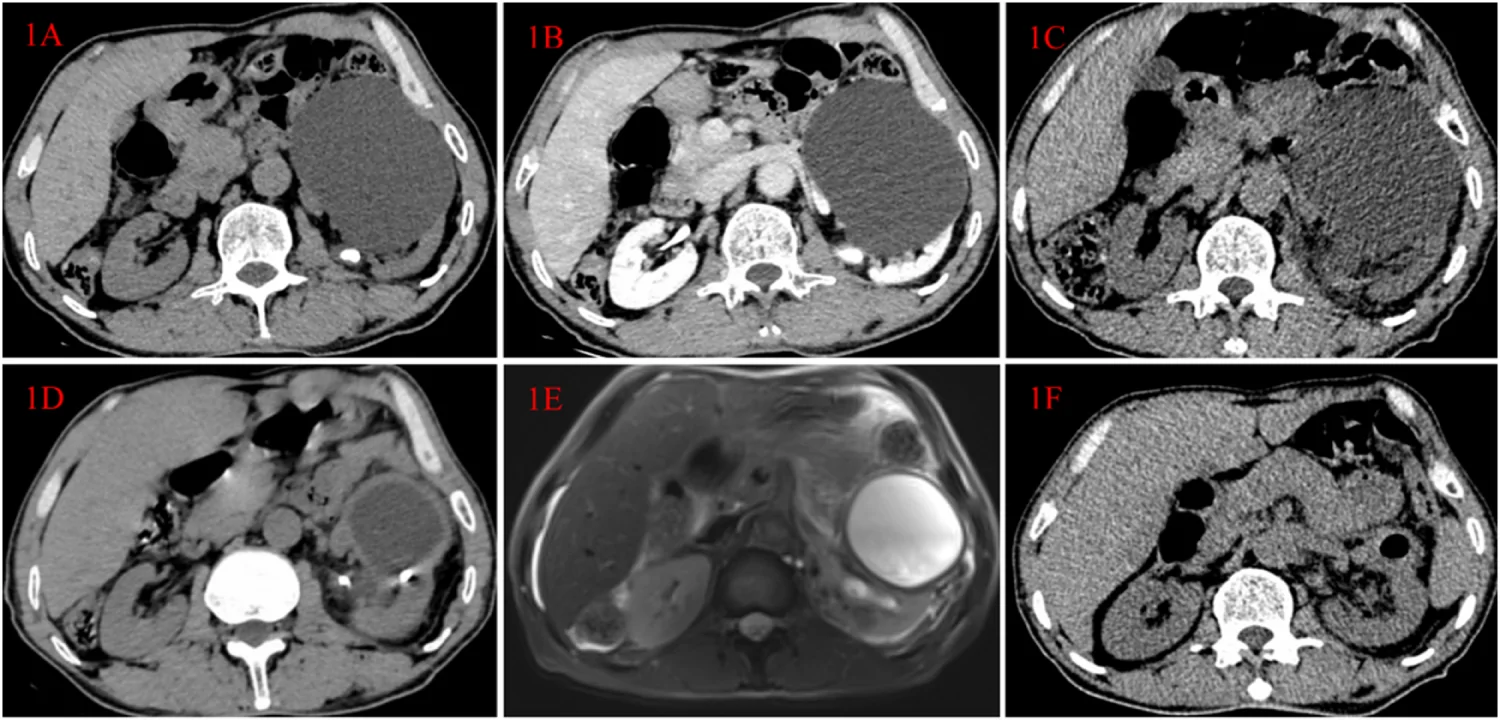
(A) On 13 November 2025, non-contrast CT on first admission showed a left renal cyst (92 × 71 mm) and a left renal calculus (10 mm). (B) Contrast-enhanced CT revealed no obvious enhancement of the lesion. (C) On 27 November 2025, the patient was admitted with renal colic. Urinary CT showed the left renal cyst with no significant change in size compared with the first admission. (D) On 5 January 2026, the patient was readmitted for flexible ureteroscopic lithotripsy. Urinary CT demonstrated that the left renal cyst had decreased in size, with a thickened wall suggestive of an infection. (E) For further characterization, MRI confirmed the presence of a left renal cyst. (F) On 1 February 2026, the patient returned for ureteral stent removal. Urinary CT showed a slight low-density lesion (18 mm) with gas accumulation in the left kidney.
